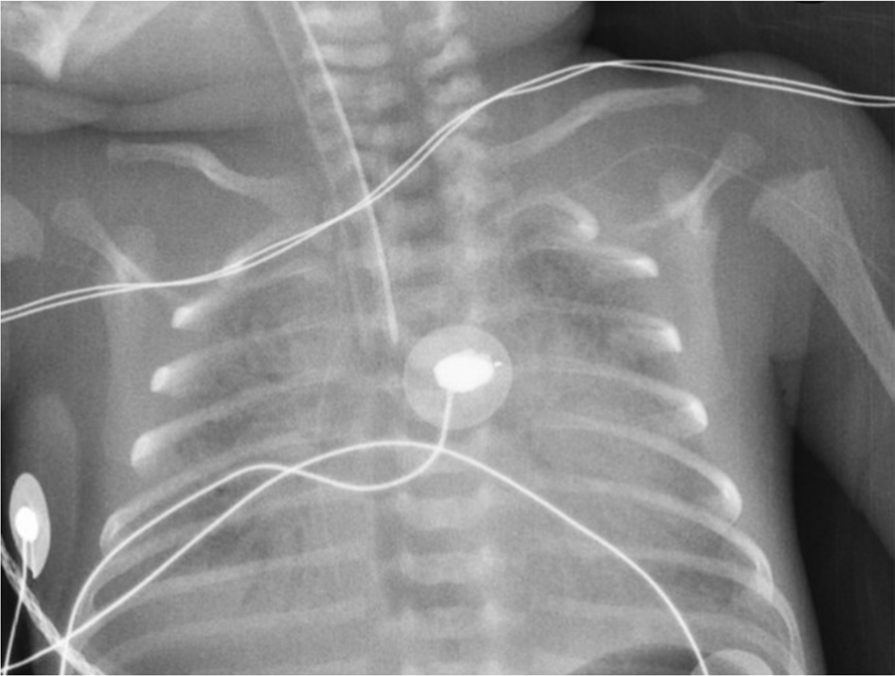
Chest X-ray, 10 h after the lipid overdose and patient intubation
